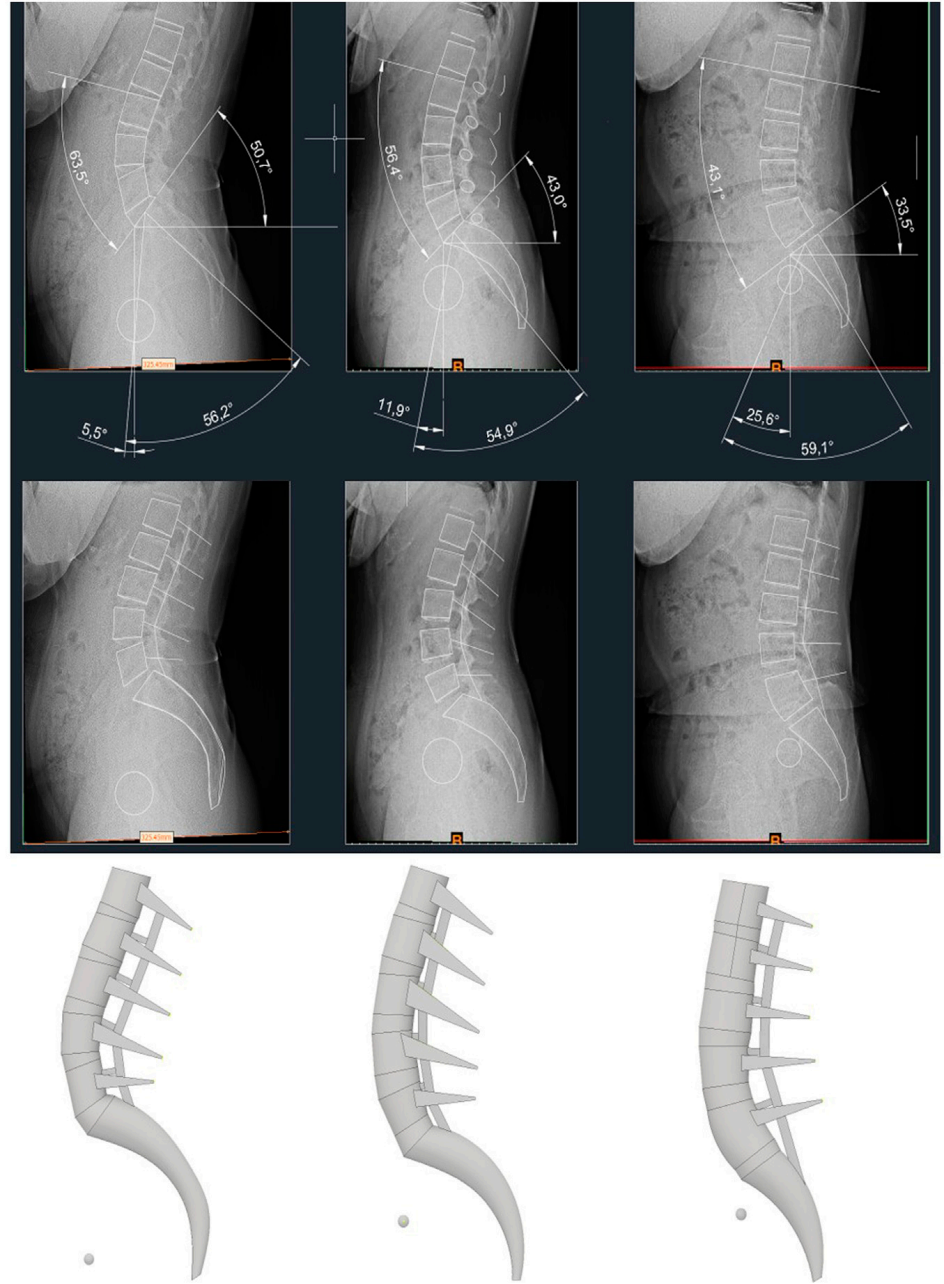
X-ray images displaying pelvic indicators of each representative model, and 3D models created based on it.
